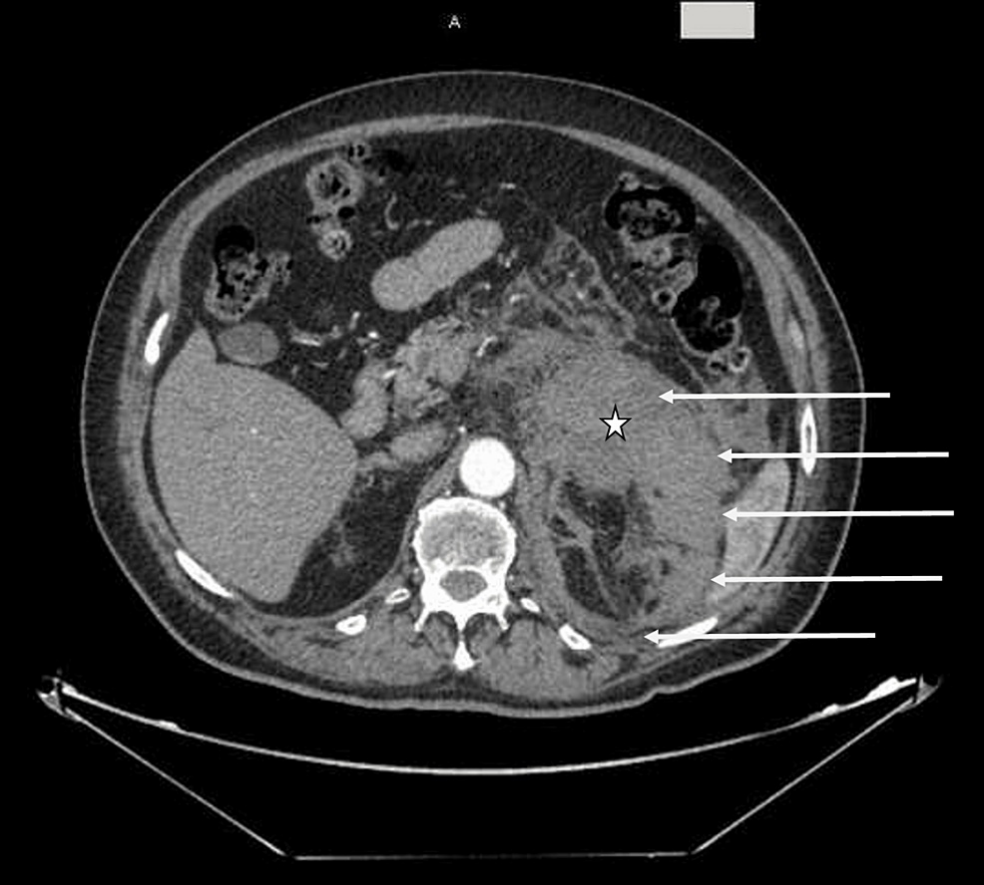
CT abdomen/pelvis showing an acute, moderate-sized left retroperitoneal hemorrhage (12 cm × 11.2 cm × 6.1 cm) centered around the left adrenal gland. The star and arrows delineate the bulk and outer borders of the adrenal hemorrhage, respectively. CT, computed tomography
